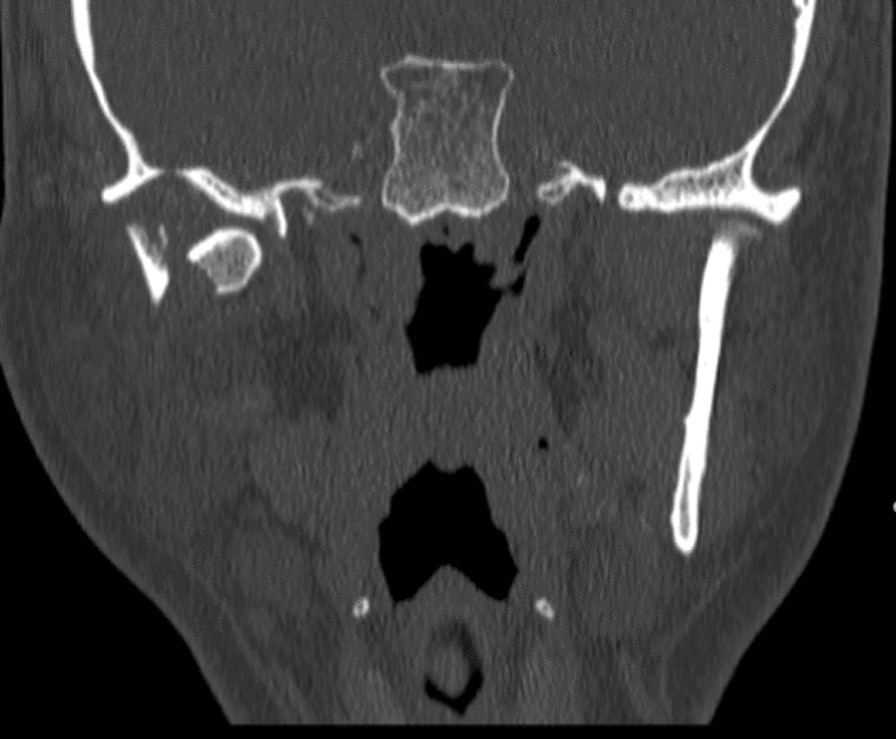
Computed tomography maxillofacial coronal view demonstrating fracture of neck of right mandible, the mandibular condyle is comminuted and displaced medially
